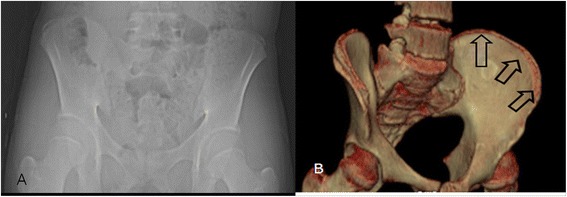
Inaccurate measurement of excursion. (A) Plain radiography performed on a 13-year-old girl; the apophysis excursion was visible covering approximately 40% of the iliac bone, and the patient was graded as Original Risser stage 2 and French Risser stage 1. (B) A 3D-CT image of the same patient; apophysis excursion was visible up to the posterior superior iliac spine and was graded as Original Risser stage 4 and French Risser stage 3.
